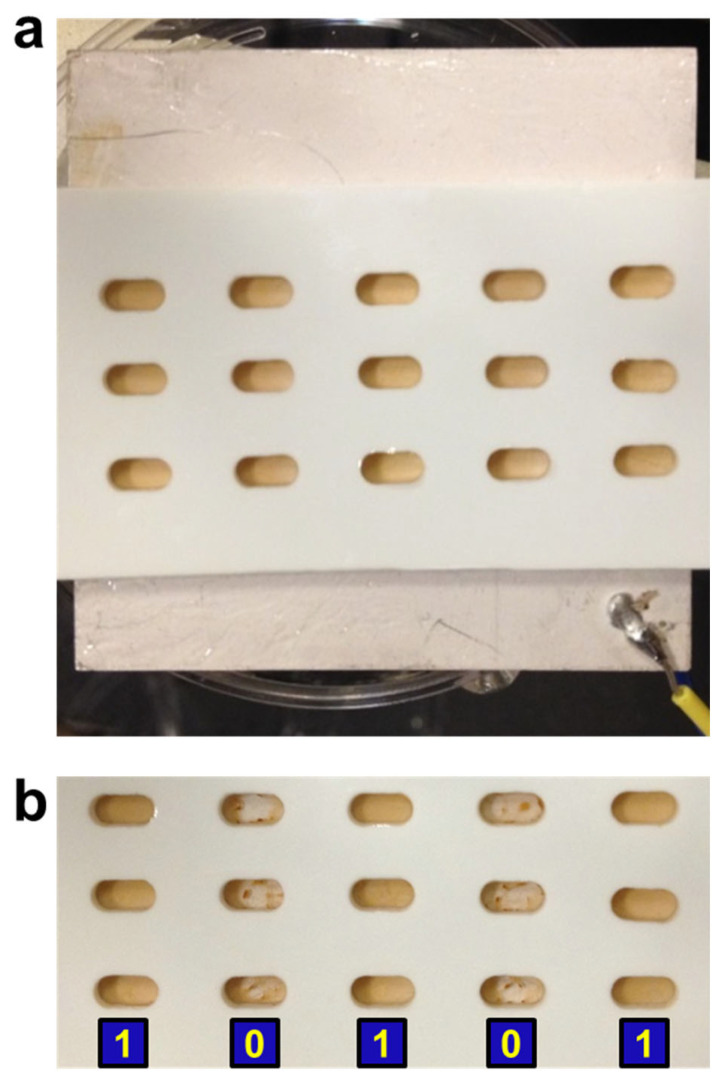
Multiplexed detection using acoustic radiation force. (a) A 15-sample plate placed on a piezo prior to applying ultrasound. (b) The sample plate after applying ultrasound. Each sample well was 4 mm long and 2 mm wide.
